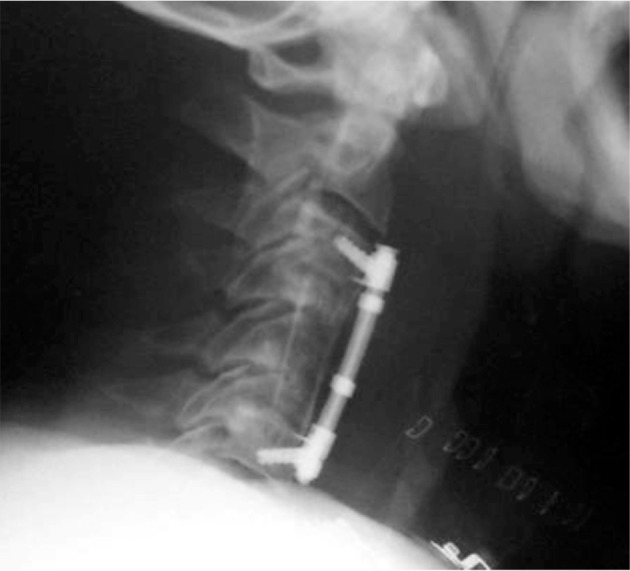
Post-Operative Lateral X-Ray Showing C3-5 Fixation With Pre- Tracheal Edema
